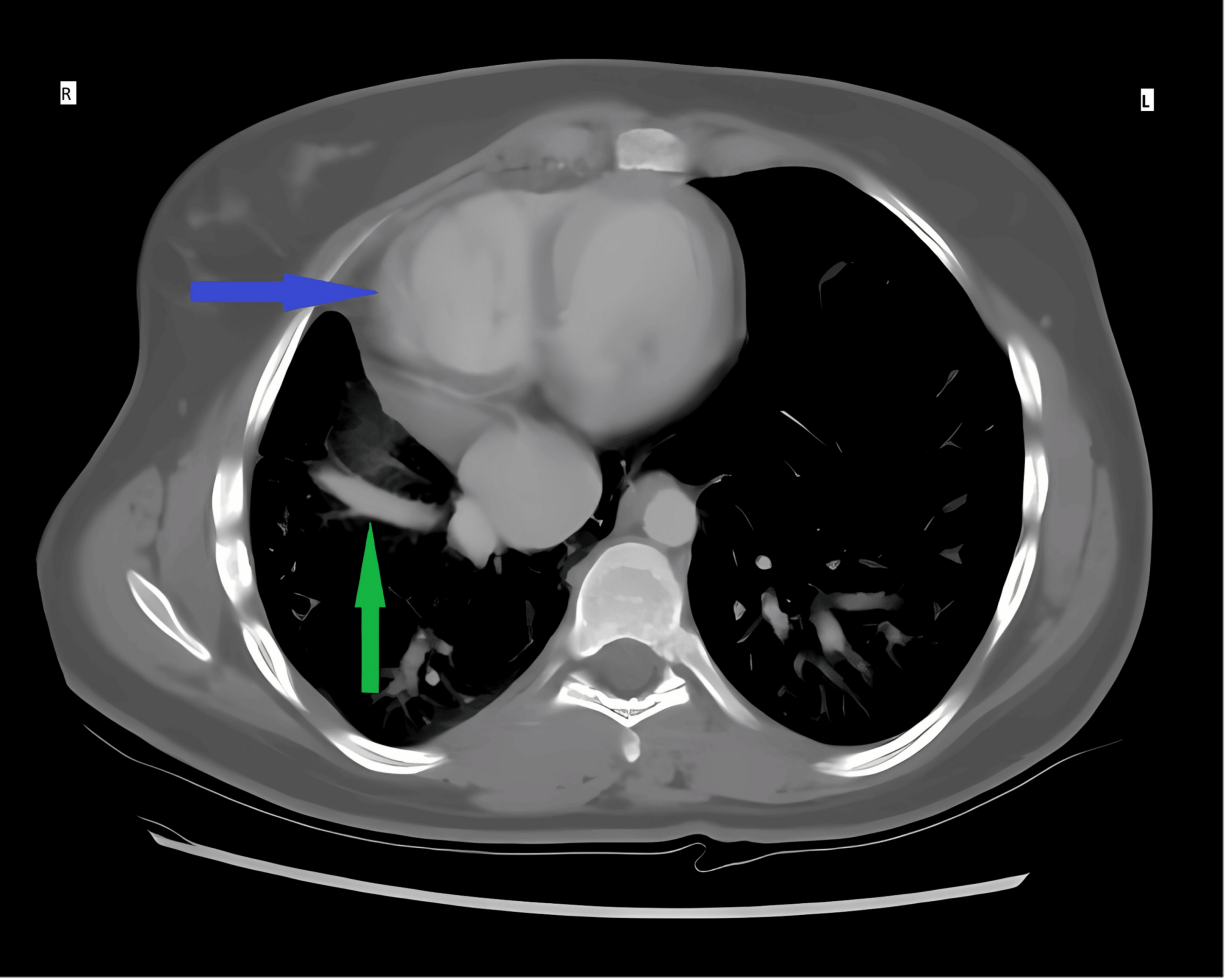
Axial CT scan (mediastinal window) The blue arrow demonstrates the mediastinal and cardiac shift to the right side (cardiac dextroposition), associated with abnormal drainage of the pulmonary veins (green arrow) into a dilated inferior vena cava. Cardiac dextroposition (rightward shift) was identified secondary to right lung hypoplasia, distinct from true dextrocardia, which involves primary cardiac malposition. Labels: R = right lung, L = left lung.
